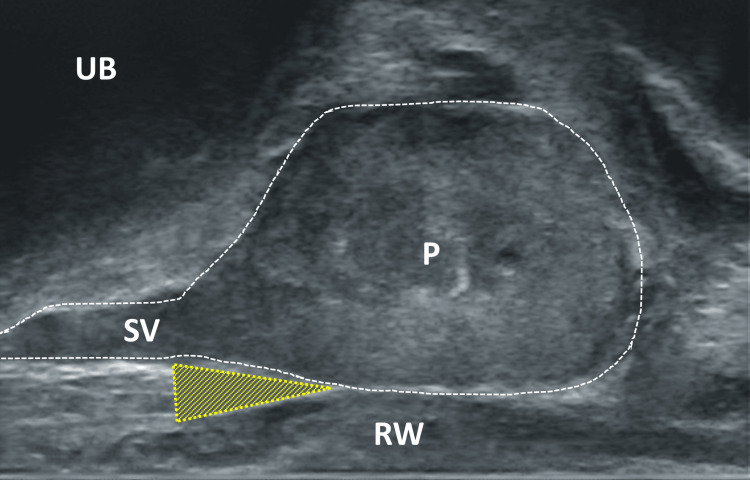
Sagittal section of the prostate, ultrasound probe slightly rotated UB urinary bladder; P prostate; SV seminal vesicle; RW rectal wall; yellow triangle: area of interest for local anesthesia between P, RW, and SV Image Credit: Toni Franz, corresponding author
